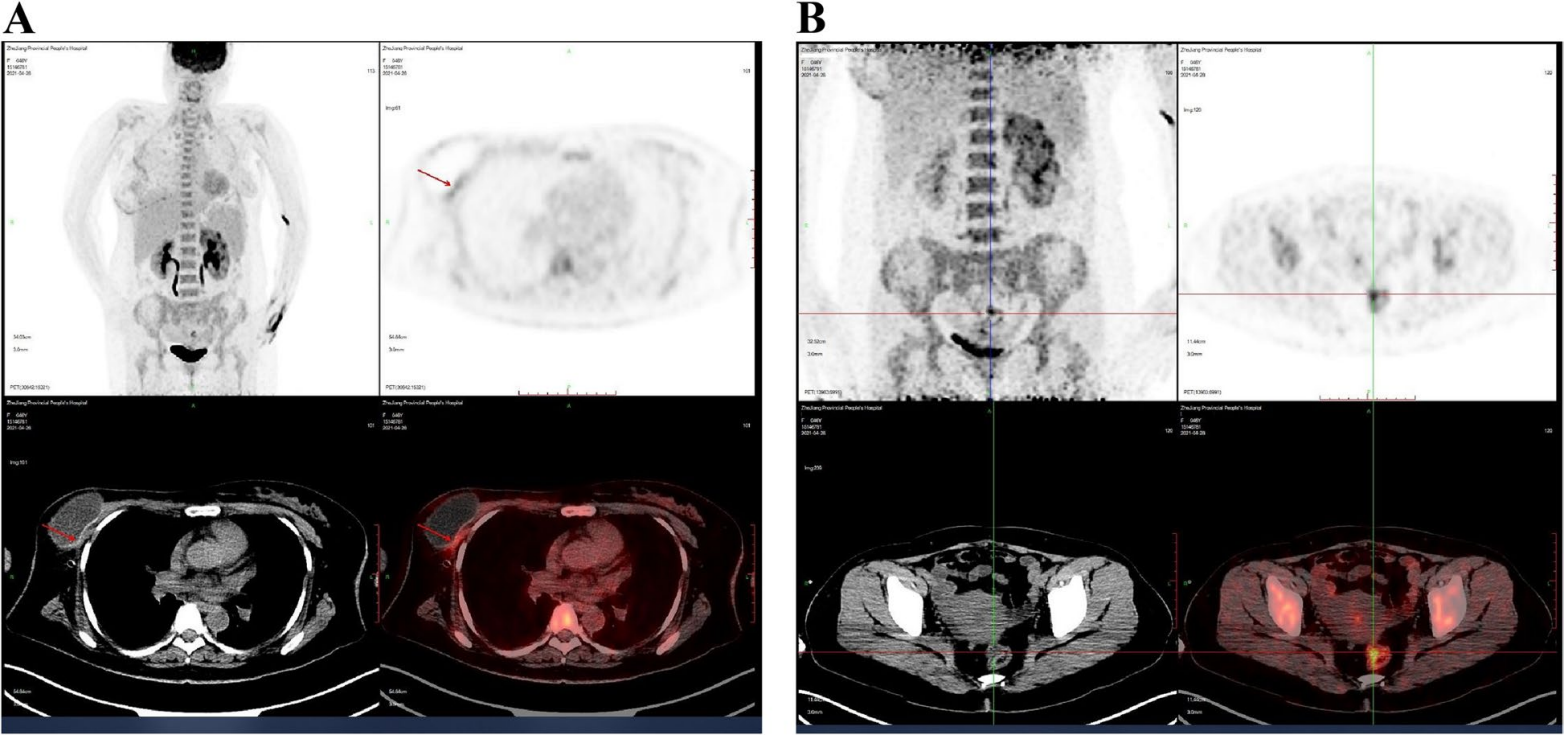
Positron emission tomography computed tomography (PET/CT) after mastectomy. A A strip soft tissue density signal was detected in the right breast surgical area, where a prosthesis was implanted. The maximum standardized uptake (SUVmax) of 18F-FDG was 5.3. B After the radical resection of colon cancer, ^18^F-FDG was increased at the anastomotic site and distal intestine with a SUVmax value of 4.4. Tracer distribution was enhanced at transverse colon, splenic flexure of colon, and rectum. The SUVmax of ^18^F-FDG was 6.6. Hysteromyoma could also be seen on the image
